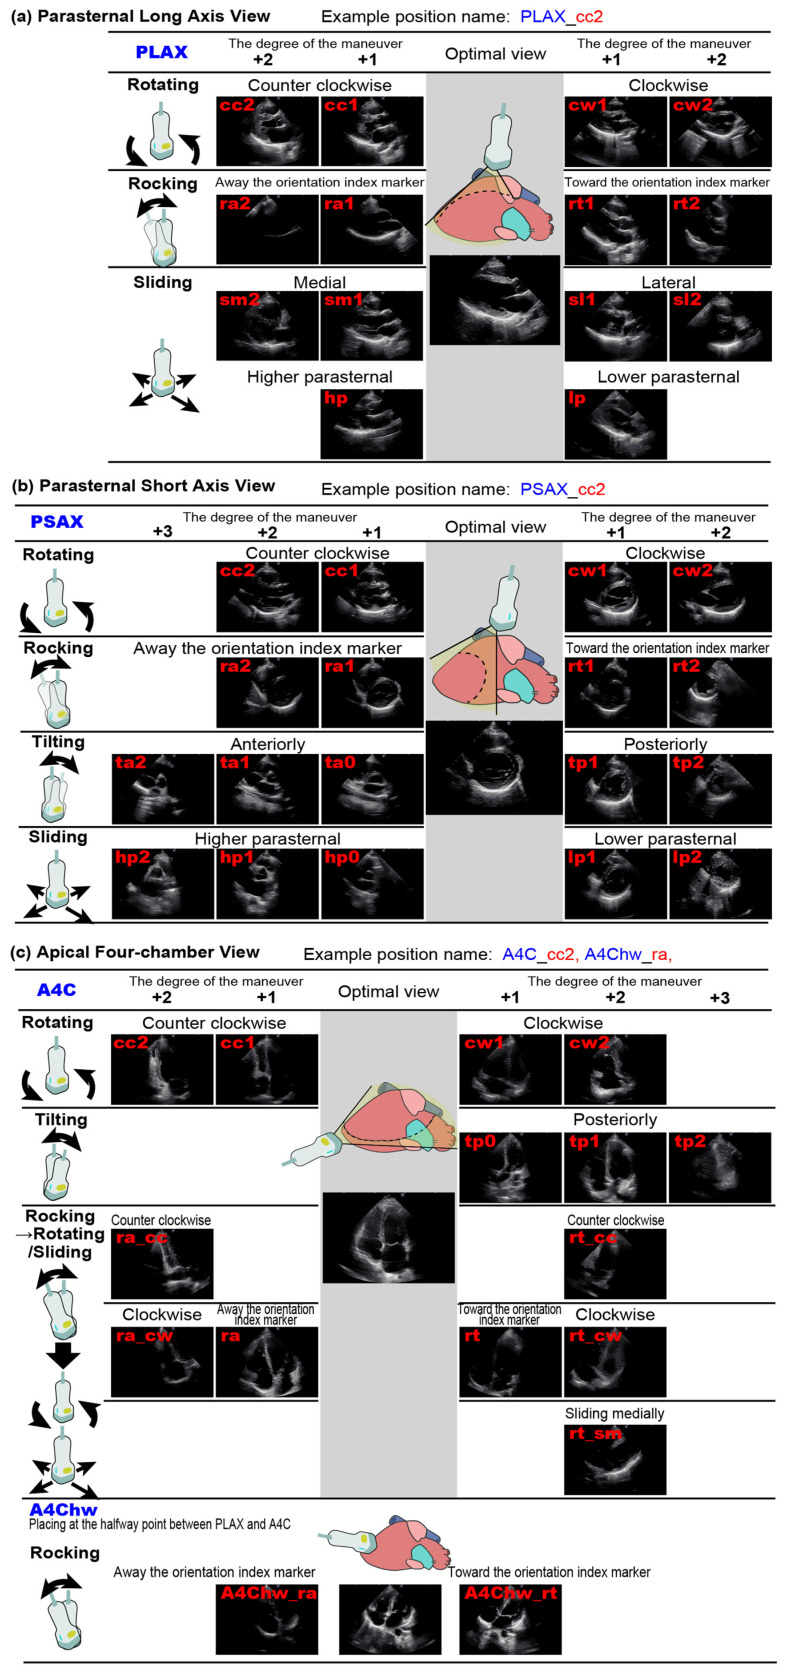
Position Labels for Images Captured in This Study. The optimal cross-sectional view for each position was defined as the starting point, and suboptimal views were obtained by applying rotating, rocking, tilting, or sliding maneuvers to the transducer. Non-optimal views were labeled by appending an underscore to the name of the optimal view, followed by an abbreviation of the maneuver (“cc” for counterclockwise rotation) and the degree of the maneuver (0, 1, or 2). (a) Parasternal long-axis view. (b) Parasternal short-axis view at the mitral valve level. (c) Apical four-chamber view.
